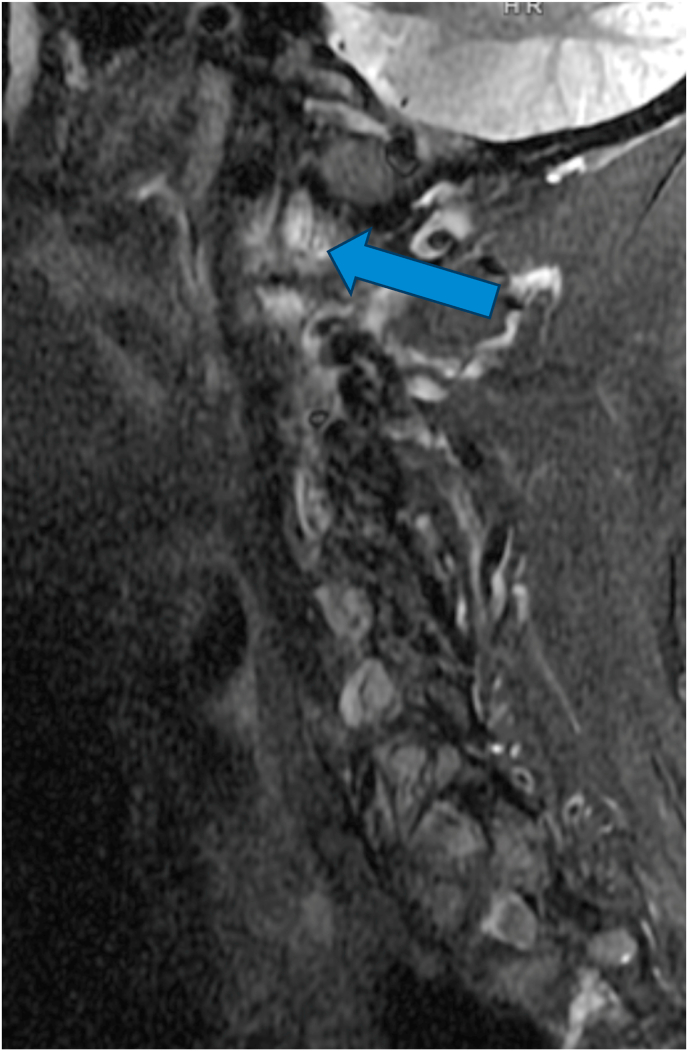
MRI showing edema in the right C1-2 joint (straight arrow).
